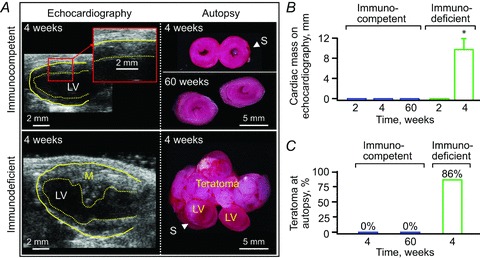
Immunocompetent status defines cell growth outcome Immunocompetent infarcted hearts were free from uncontrolled growth following iPS cell implantation as documented *in vivo* (echocardiography; *A* and *B*) and on autopsy (*A* and *C*) during the 60-week-long follow-up, in contrast to teratoma formation observed in immunodeficient hosts. In *A*: M, mass; LV, left ventricle; S, suture for coronary ligation. In *B*, data represent means ± SEM (*n*= 8 immunocompetent hearts: *n*= 7 immunodeficient hosts); P* < 0.05 *versus* immunocompetent.
